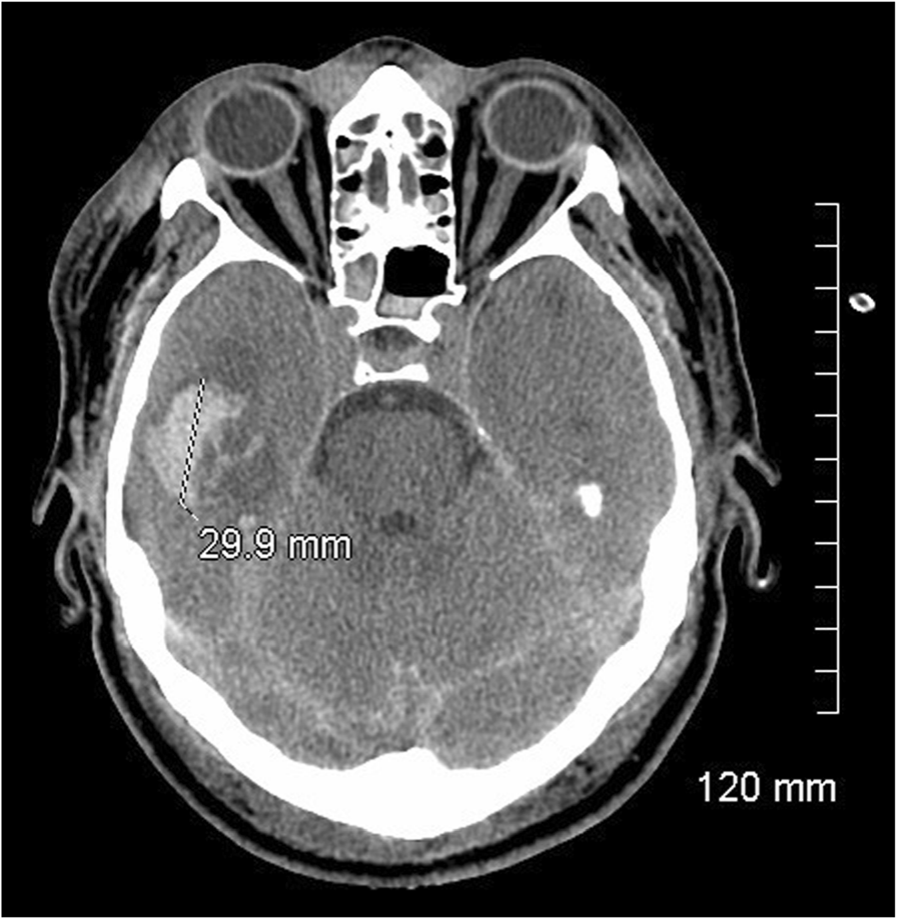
CT scan at admission. Initial CT scan taken at admission, showing right temporal parenchymal hematoma, diffuse subarachnoid haemorrhage in the left hemisphere, and diffuse axonal injury
